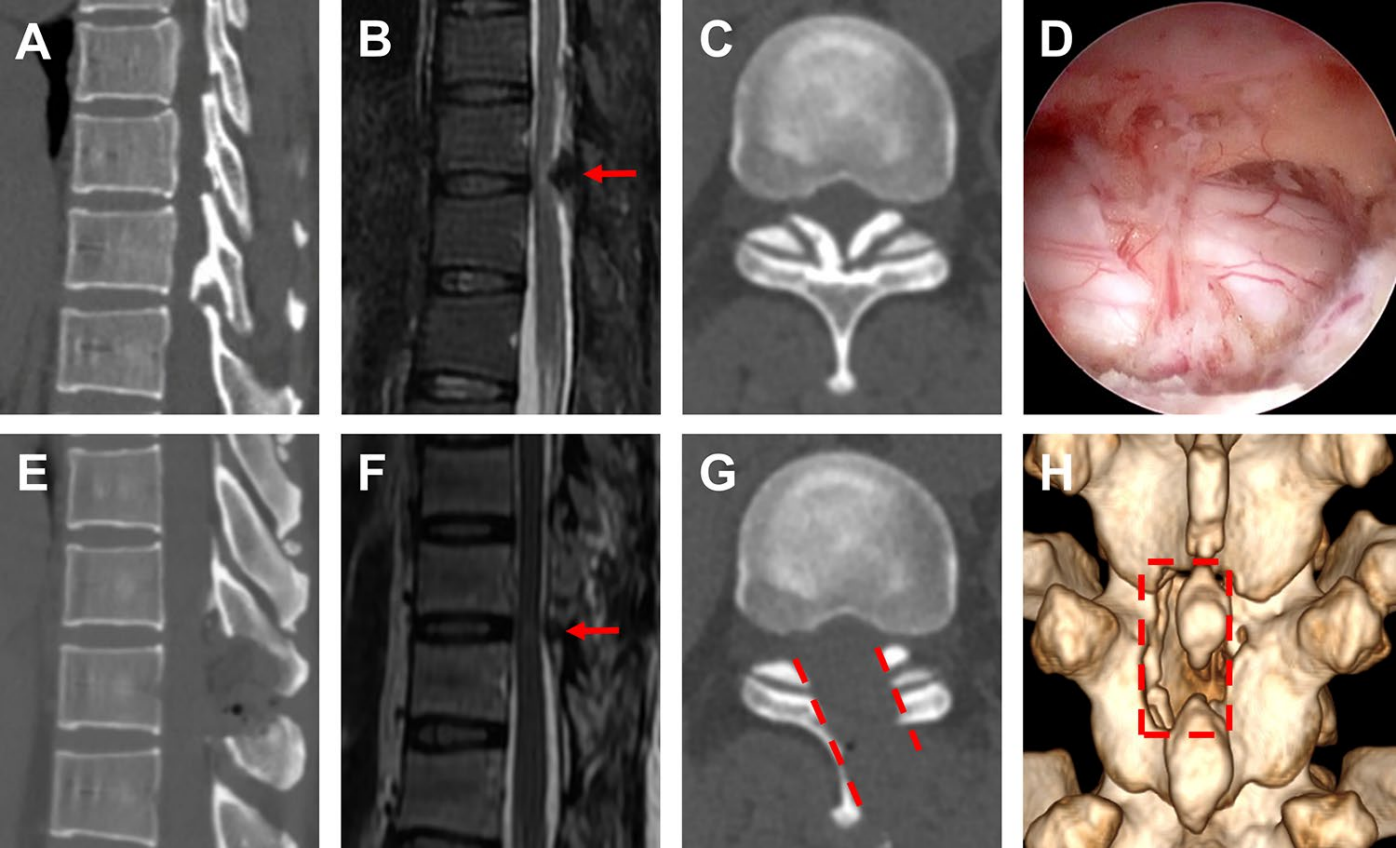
Single segment TOLF treated with OSE procedure. (A–C) Preoperative sagittal, axial CT and MRI images of TOLF, and the ossification lesion compressed the spinal cord at T10/11 (As shown by the red arrow). (D) The view of decompression of spinal cord during operation on endoscope. (E–G) Postoperative sagittal, axial CT and MRI images, demonstrated a complete remove of ossification lesion (As shown by the red arrow and dashed). (H) Postoperative 3D-CT image showed the range of unilateral laminectomy at T10 and T11 (As shown in the red dashed region), bilateral decompression of spinal cord through unilateral approach.
